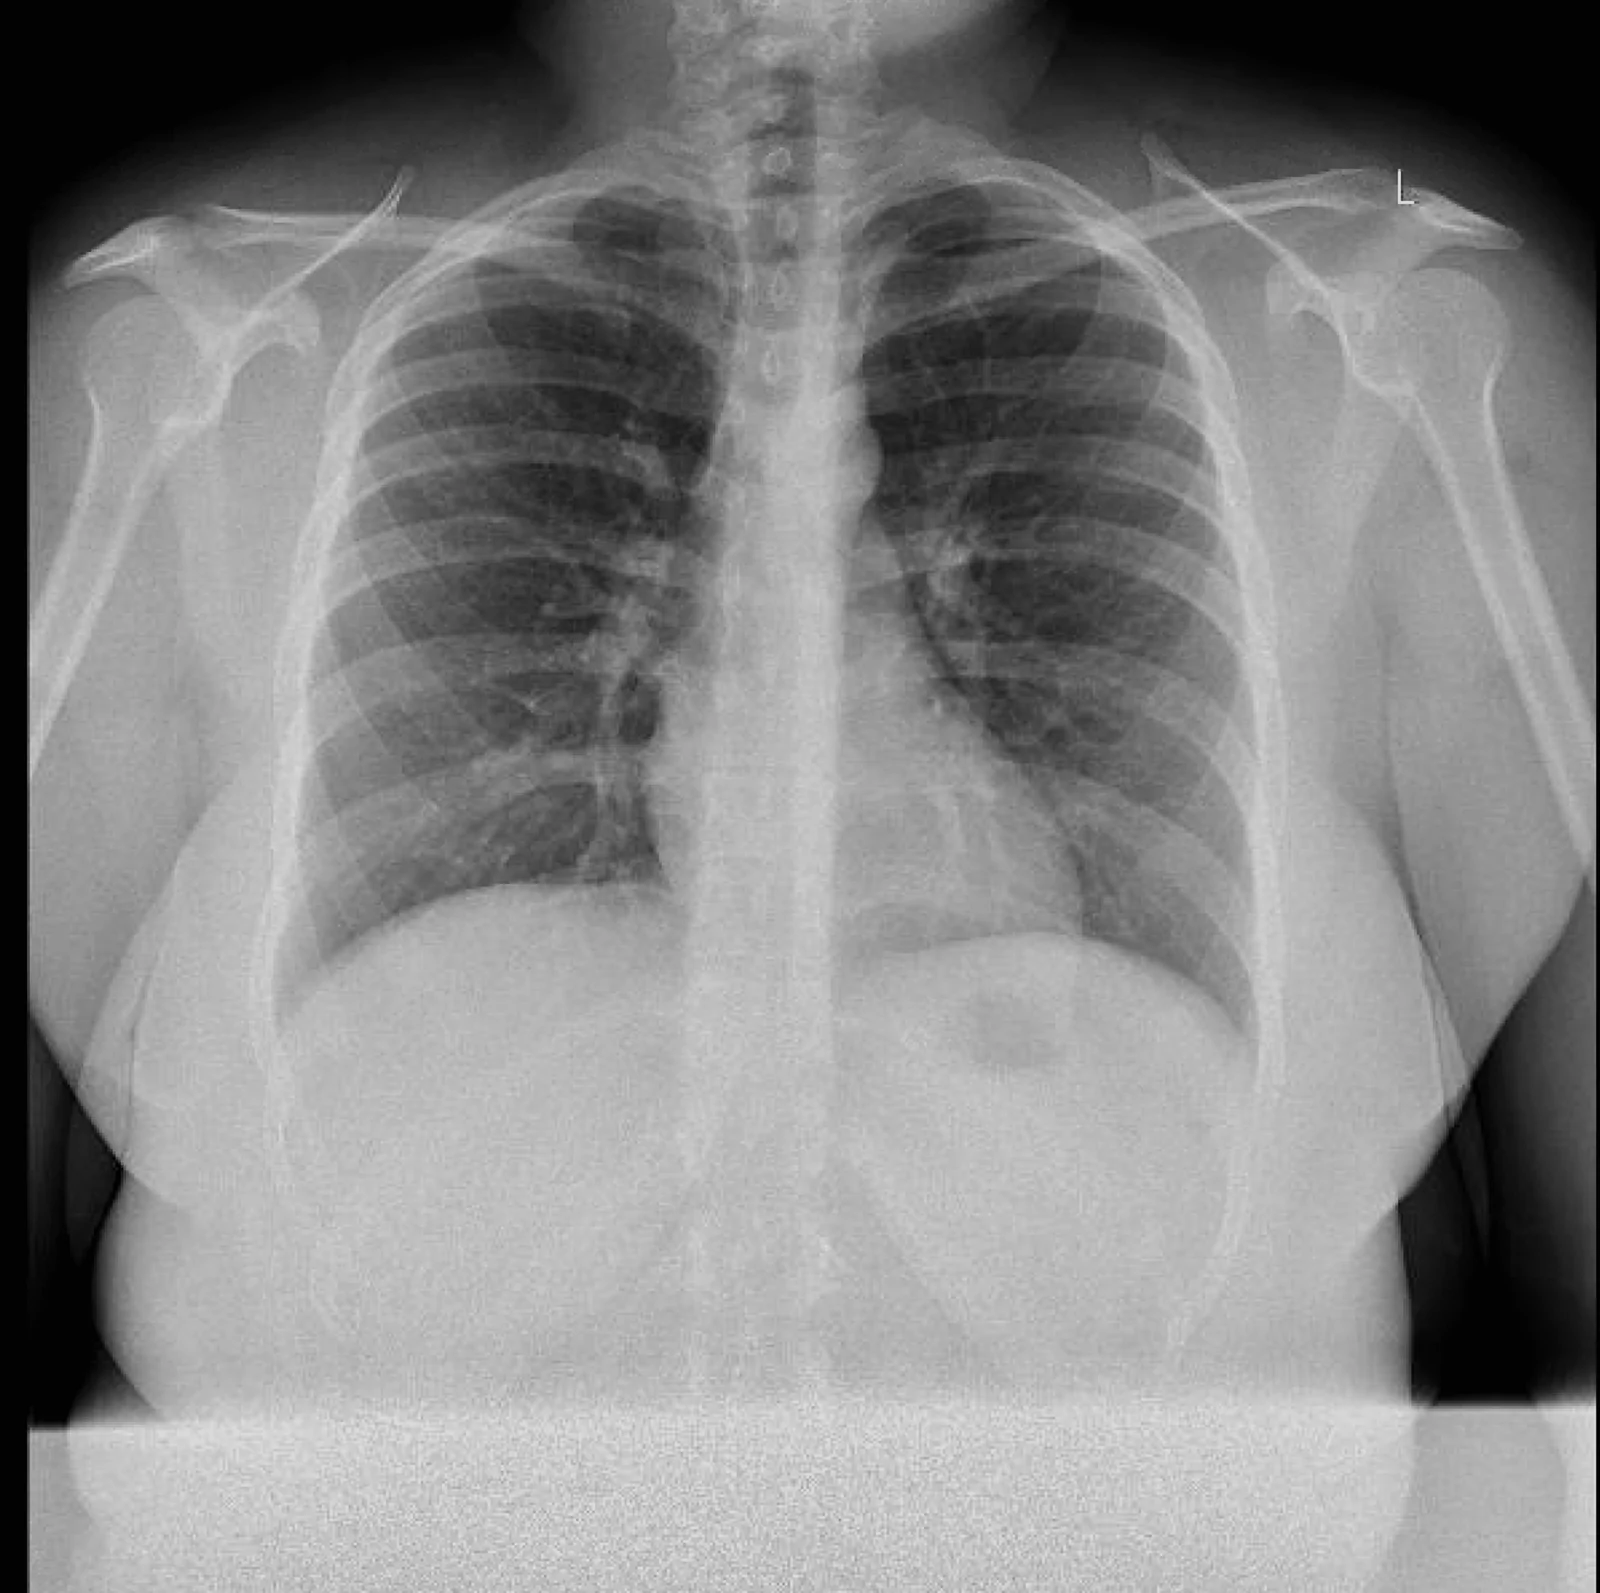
Follow-up posteroanterior (PA) chest X-ray demonstrating interval improvement of the pneumomediastinum, with a small residual right paratracheal pneumomediastinum. No pneumothorax is identified.
